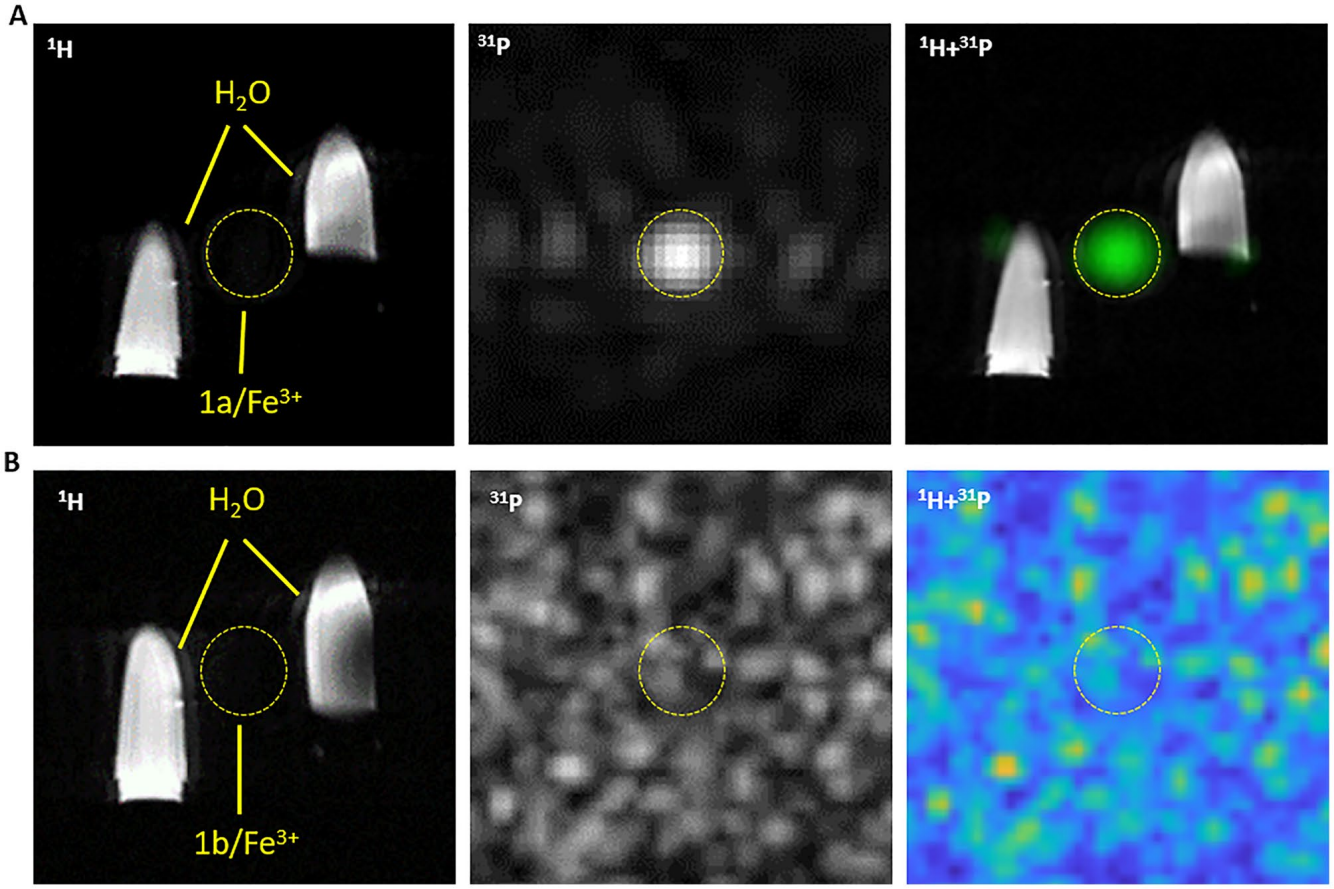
^1^H MRI (on the left), ^31^P MR CSI (in the middle) and overlapped ^1^H MRI/^31^P MR CSI measurements (on the right) of vials with water on the sides (controls) and a vial with a solution of (A) 1a/Fe^3+^ and (B) 1b/Fe^3+^ complexes between them.
